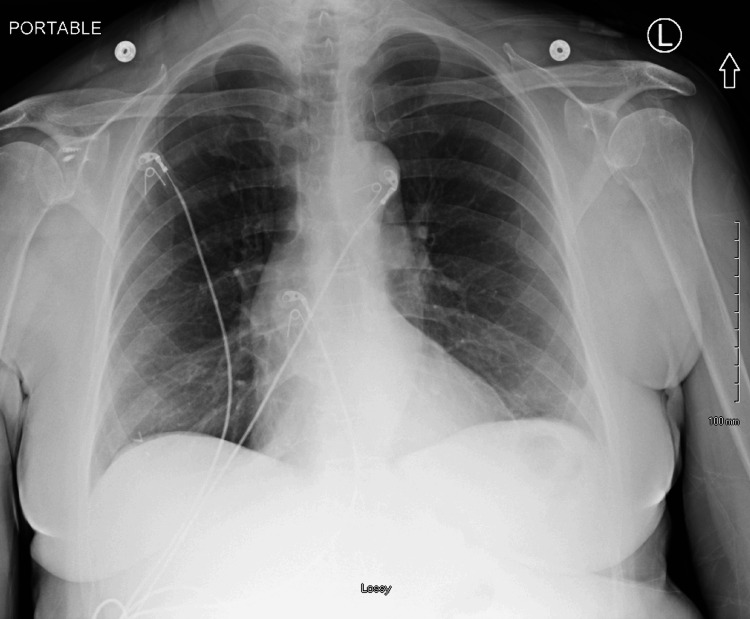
Portable AP Chest X-ray showing no acute pulmonary findings. Mild cardiomegaly is noted.
